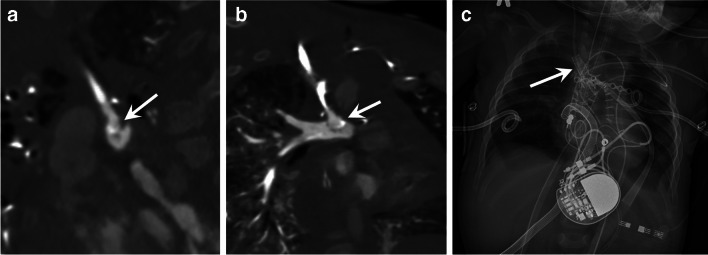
A 9-month-old boy with double outlet right ventricle, surgically repaired with a partial cavopulmonary shunt (Glenn procedure) and extracorporeal membrane oxygenation (ECMO) treatment. The patient was hemodynamically unstable. Contrast-enhanced pulmonary computed tomography angiography with multiplanar reconstructions reformatted images in the sagittal (a) and coronal (b) planes show a linear endoluminal lesion consistent with pulmonary artery dissection (*arrows*). c Postoperative anteroposterior chest radiograph showing endotracheal tube, ventricular assist cannulae, a pacemaker and pleural drainage catheters, and a stent placed at the Glenn anastomosis (*arrow*)
